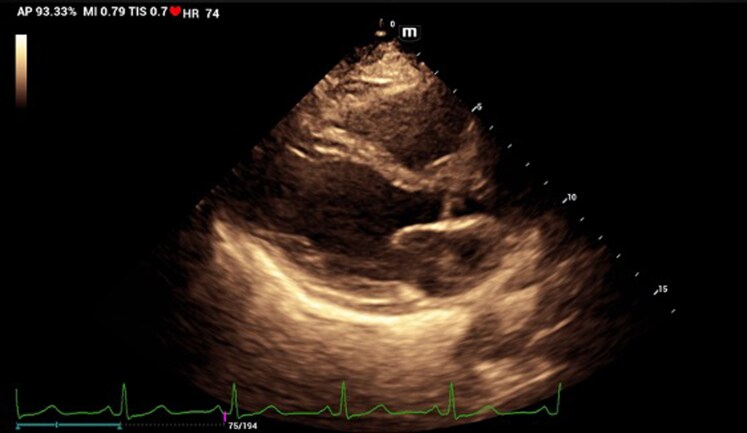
Echocardiographic evaluation showed no pericardial effusion.
